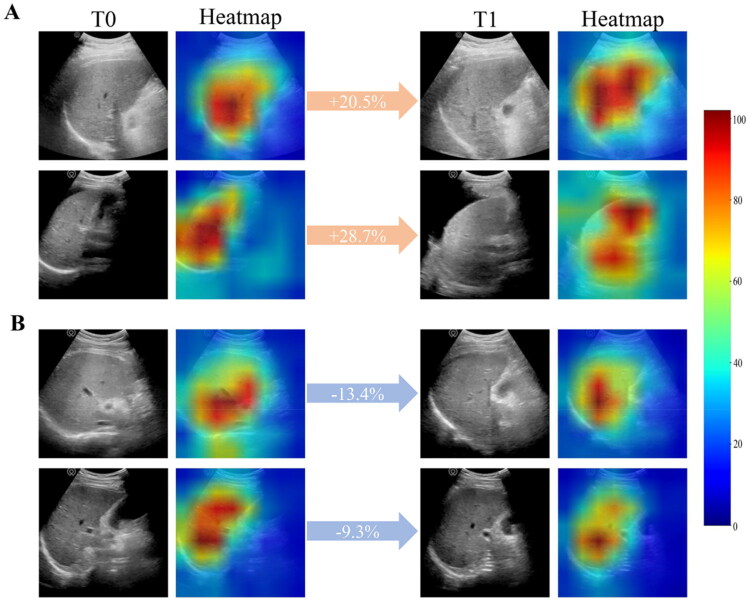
Siamese U-Net visualization and interpretation. Color-coded heatmaps from key channels at the output of the Siamese U-Net, overlaid with corresponding ultrasound images at T0 and T1 time points for four accurately predicted patients. (A) For two non-survivors, T1 heatmap activation intensity percentages were 20.5 and 28.7% higher than T0, with increased activation predominantly in liver texture, portal veins, major vasculature, and ascites. (B) In contrast, T1 heatmap activation intensity percentages for two survivors decreased by 13.4 and 9.3% compared to T0, with reduced activation across hepatic parenchyma and portal venous systems.
